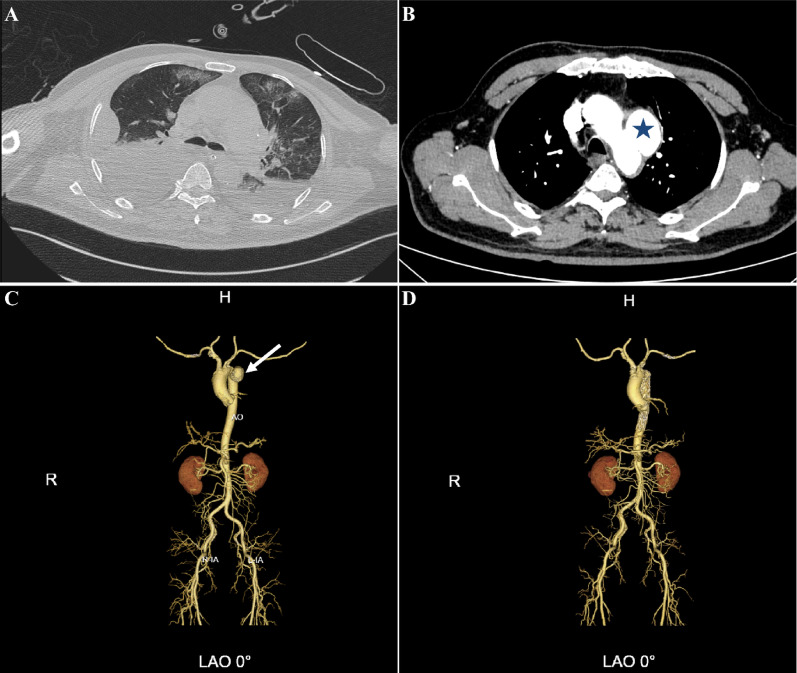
A, Chest CT shows severe lung infection and pleural effusion; B, The blue asterisk marks the location of the aortic arch pseudoaneurysm; C, Three dimensional reconstruction CT showed that the pseudoaneurysm was located in the aortic arch; D, Endovascular aortic intervention isolated the pseudoaneurysm.
